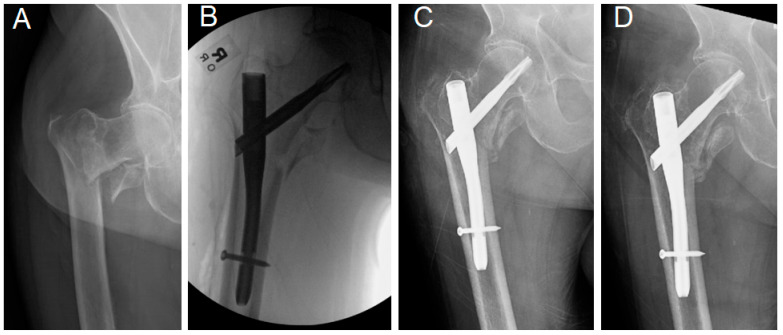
(A) Preoperative, (B) Immediate postoperative plain radiographs of 1 95-year-old male with an intertrochanteric fracture. Note the fractured/incompetent lateral wall. Note also that the lag device does not perfectly engage the lateral wall. (C) 8-week and (D) 16-week follow-up radiographs showing nail toggling and varus fracture collapse.
